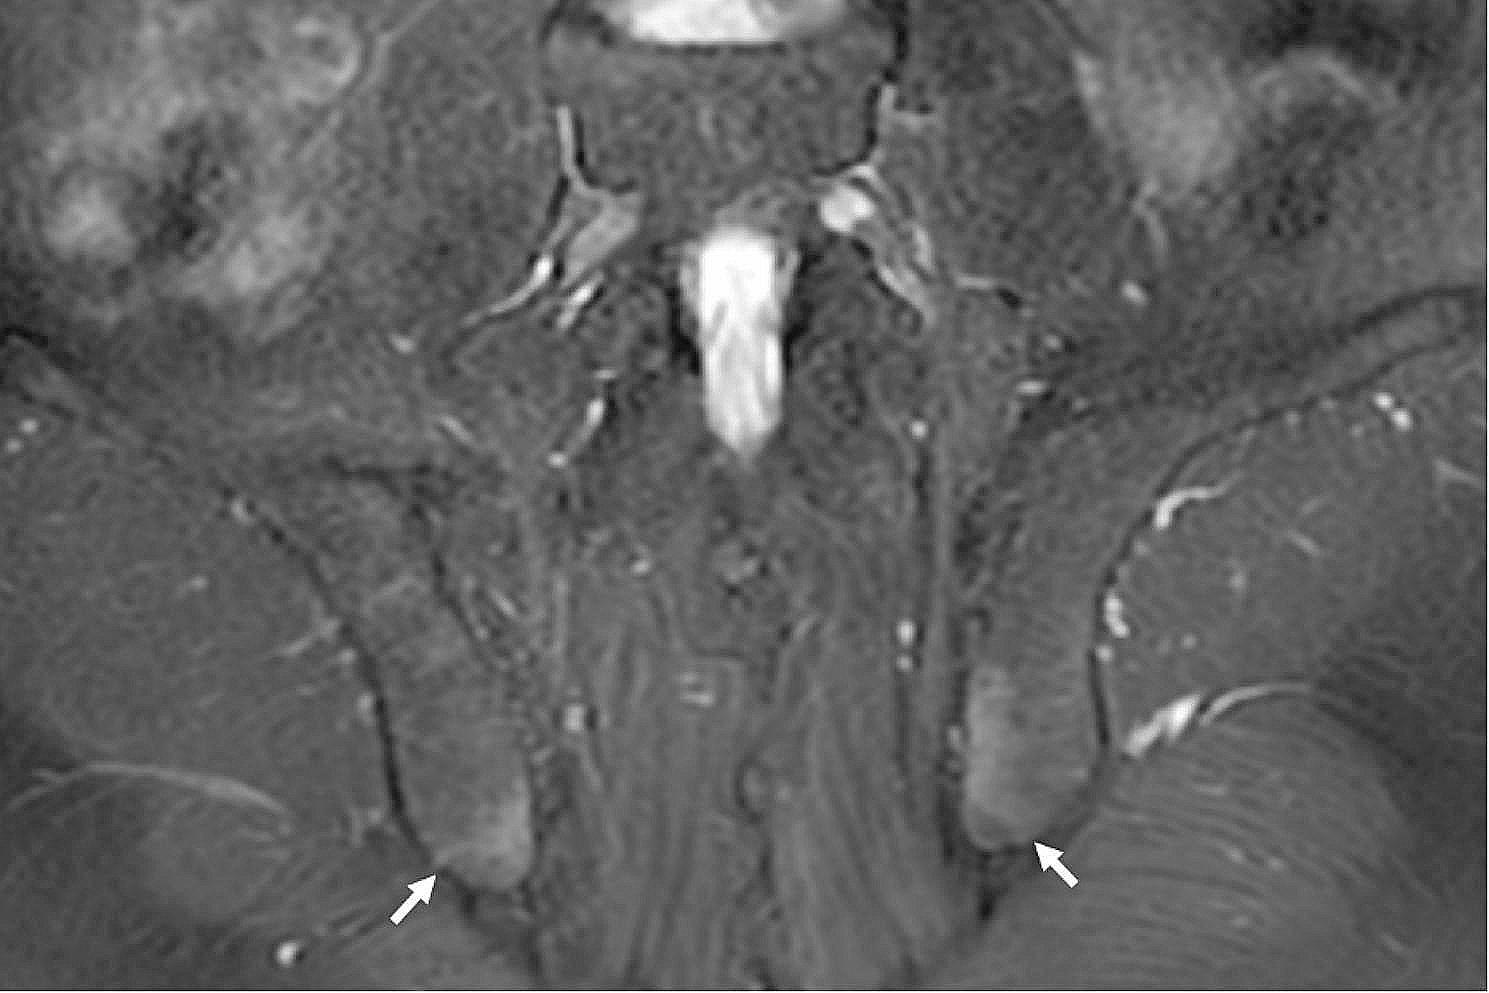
Enthesitis in a 37-year-old male with axial spondyloarthritis. Coronal oblique T2-FS MR images exhibited bilateral posterior sacroiliac ligament enthesitis at the inferior iliac quadrant (arrow)
